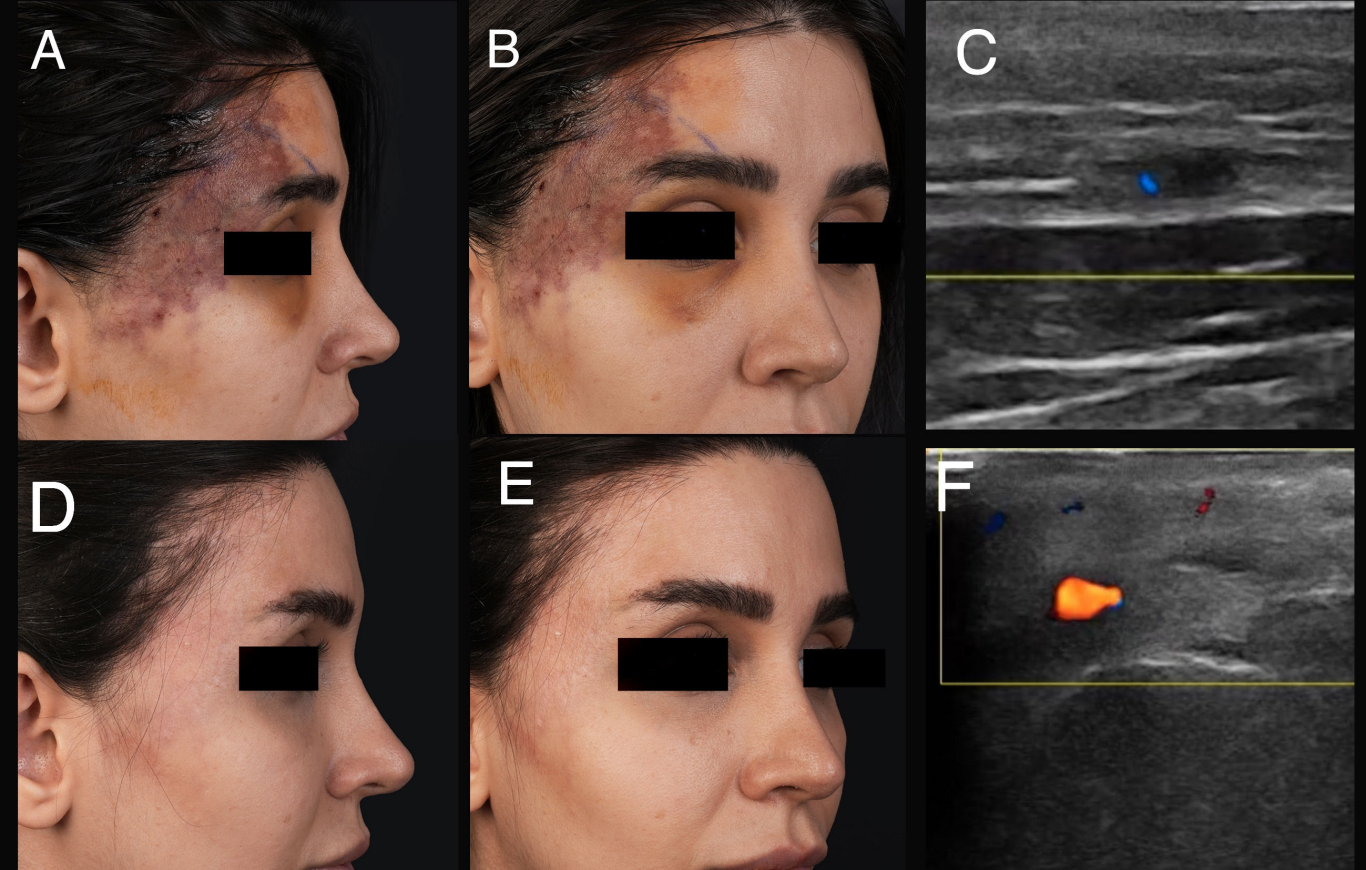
A 31-year-old female experienced ischemia following an injection of 0.5 cc HA filler. (A,B) A clinical presentation after 119 h of filler injection. (C) An ultrasound examination before treatment (Involvement of frontal branch of superficial temporal artery). (D,E) Improvement of ischemia a month after the “THIS and FAT” protocol. (F) An ultrasound examination after treatment (arterial flow return to normal).
